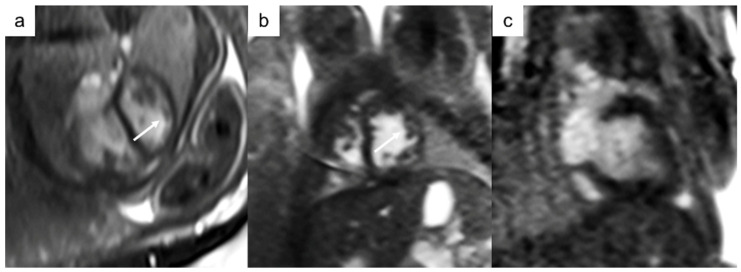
Thirty-week gestational age fetus with suspected left ventricular (LV) non-compaction syndrome at fetal echocardiography. fCMR confirmed the hypertrabeculation of the LV myocardium (white arrows) together with an increased cardio-thoracic ratio: (a) 4-chamber view, (b) short-axis view, mid-ventricular planes, and (c) 2-chamber view.
